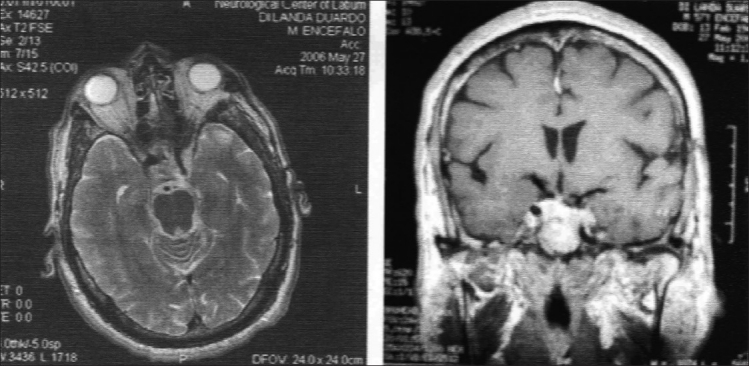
Same patient as in Figures 1 and 3. Two-year postoperative MRI. T2 axial. a) Post-contrast T1 coronal; b) Scans show no recurrence of the tumor
